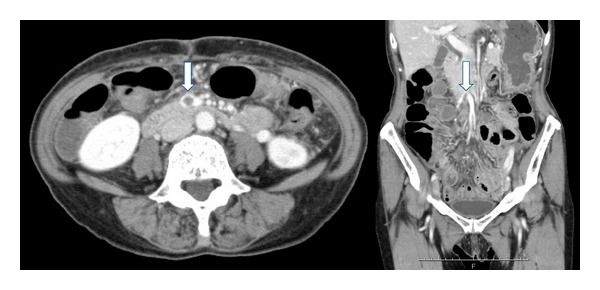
Contrast enhanced computed tomographic scan (axial and coronal views) of the abdomen demonstrated a filling defect in the superior mesenteric vein (arrow), suggesting thrombus.
